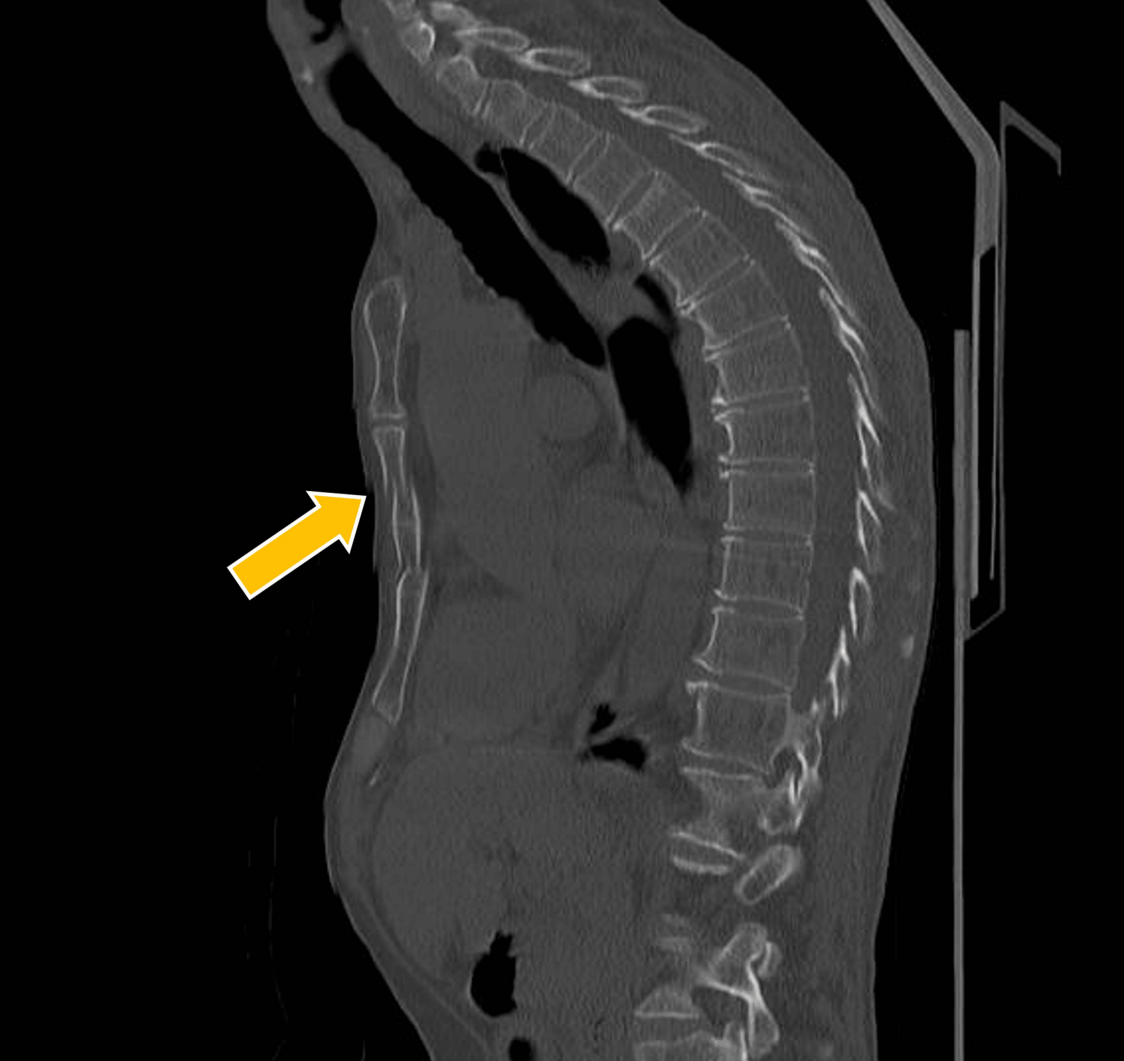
Preoperative chest computed tomography. The orange arrow indicates fracture of the sternum
